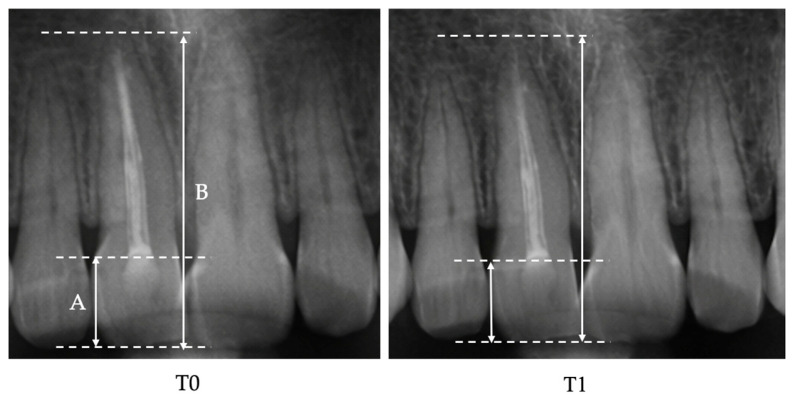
Measurement method for root length assessment in an endodontically treated tooth. A. Crown length measured as the longest distance between the incisal edge and the cemento-enamel junction (CEJ). B. Total tooth length measured as the longest inciso-apical distance from the incisal edge to the radiographic root apex.
